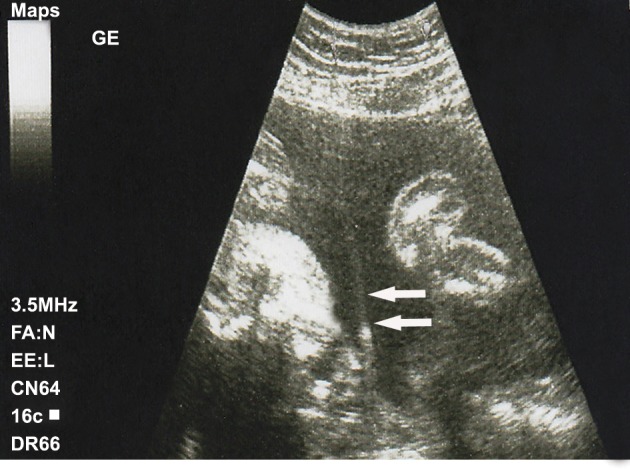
Ultrasonographic image of the patient at second admission, interpreted as amniotic band.
